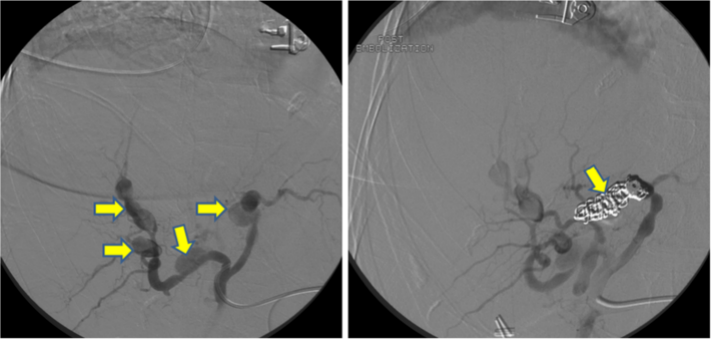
Traditional x-ray angiography may still be employed in most centers to diagnose polyarteritis nodosa involving the visceral arteries, such as the focal, segmental aneurysms (left panel, up to 4.5 cm in diameter) of the hepatic artery seen in this 77 year-old woman with acute abdominal pain, syncope and bleeding within the liver parenchyma by CT. The angiographic appearance was consistent with PAN. Coil embolization (right panel) was performed with resolution of bleeding. Images courtesy Dr. Leslie Cooper.
